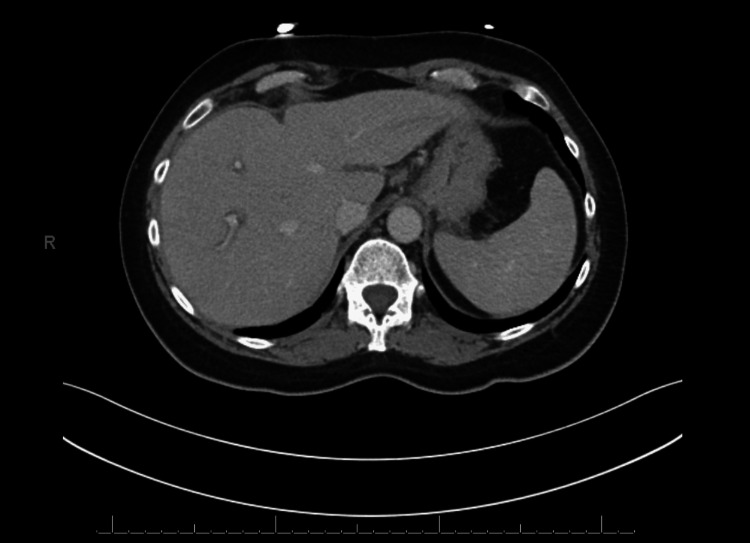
Computed tomography of the abdomen and pelvis without acute hepatobiliary abnormality Axial computed tomography image of the abdomen and pelvis demonstrating no acute hepatic abnormality and no biliary ductal dilatation, supporting the absence of an obstructive or structural cause of transaminitis.
